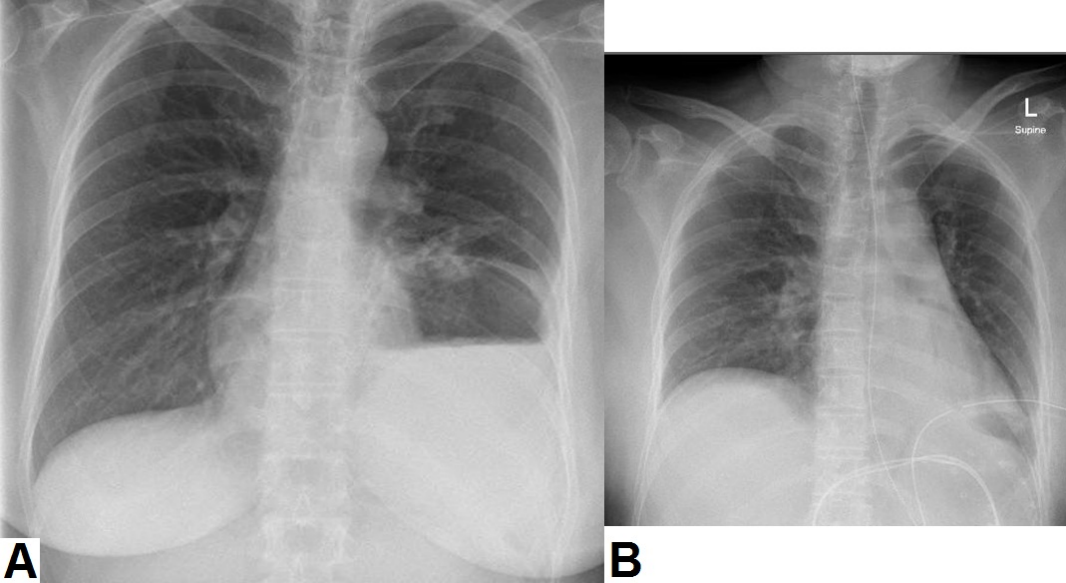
Plain chest radiographs of the patient (A) Plain chest radiograph showing a raised left hemi-diaphragm with visualization of a gastric bubble within the left hemi-thorax. (B) Postoperative radiograph demonstrates the presence of a surgical drain and nasogastric tube, and reduction in the level of the left hemi-diaphragm.
